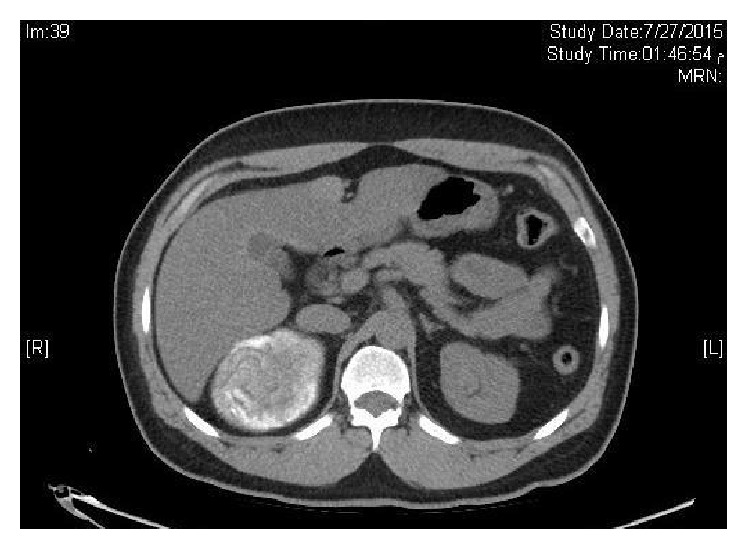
CT scan abdomen showing (R) adrenal mass.
